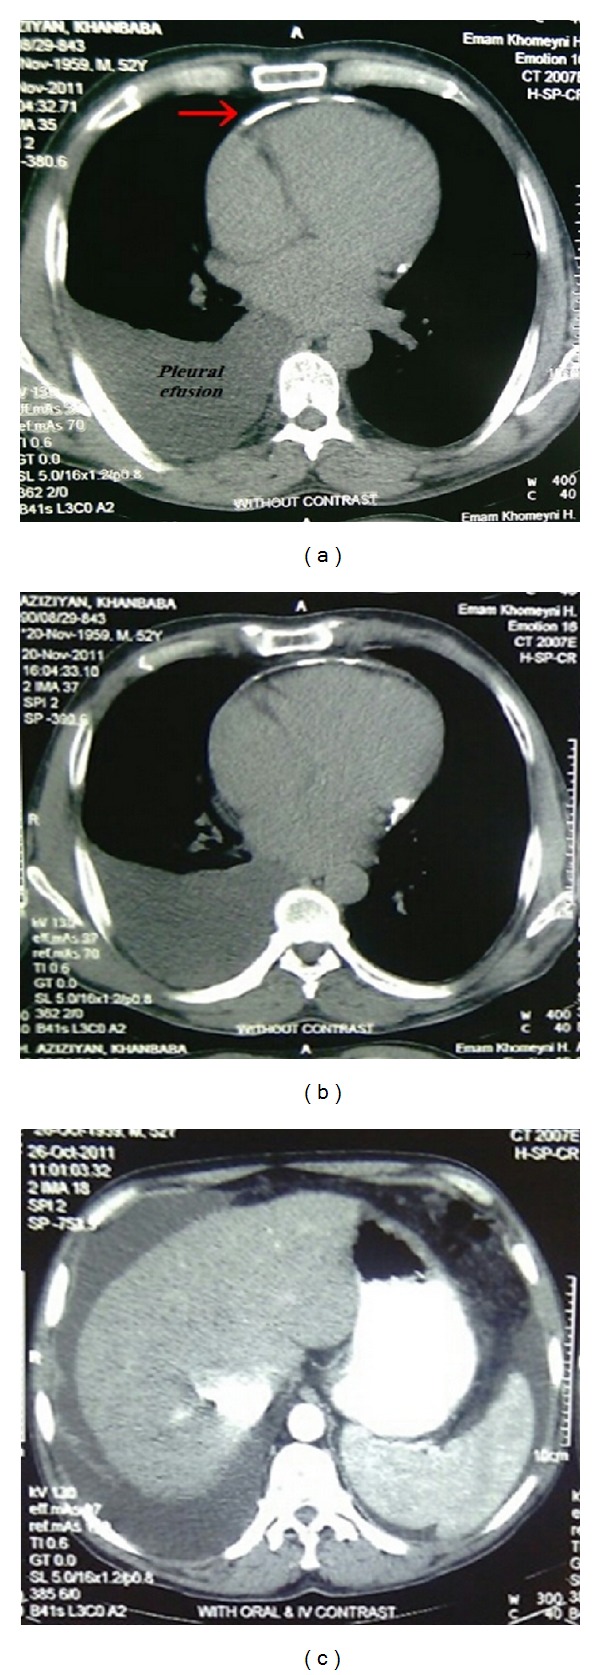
Chest CT showing pleural effusion, cardiac calcification, ascites, and IVC dilation.
